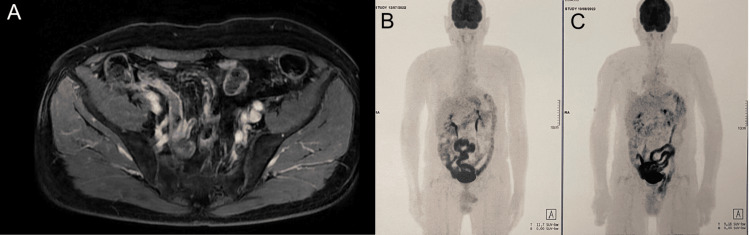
MRI and PET-CT scans performed in 2023 show no evidence of disease MRI: magnetic resonance imaging; PET-CT: positron emission tomography-computed tomography
